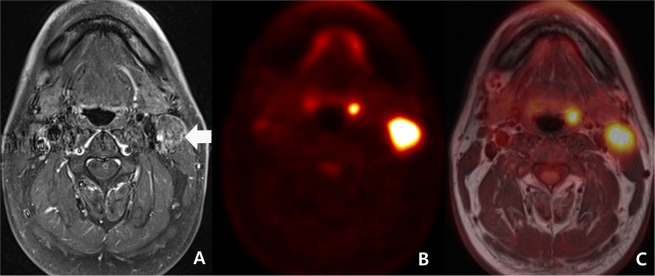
Initial work-up of a 54-year-old man with left palatine tonsillar cancer (discordant diagnosis) and ipsilateral cervical lymph node metastasis (malignant concordant diagnosis). (A) A transverse fat-suppression contrast enhanced MRI revealed enhancing lesion at left cervical chain (arrow). A neuroradiologist scored the lesion as 5 (definitely malignant). However, he failed to reveal primary focus in the scan. (B) As PET reveals hypermetabolism (maximal SUV: 12.3) at the left cervical chain, a nuclear medicine specialist scored the lesion as 5 (definitely malignant). In addition, because PET shows a small hypermetabolic lesion at left palatine tonsil (maximal SUV: 8.3), he scored the lesion as 4 (probably malignant). (C) A consensus score of PET/MRI for left cervical lesion was 5 (definitely malignant) and a consensus score of the left palatine tonsillar lesion was 4 (probably malignant). Both left cervical chain lesion and left palatine tonsillar lesion were proved to be squamous cell carcinoma.
